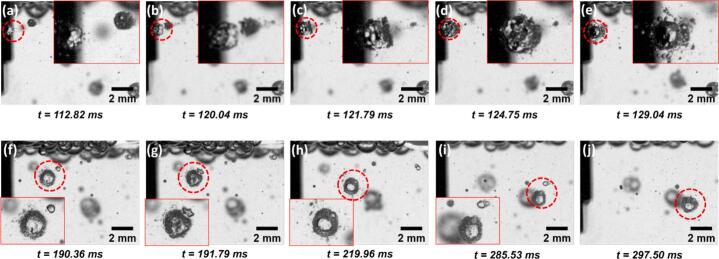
Bubble coalescence in TX-100 solution at 100 % CMC during UST. The inset images in the figures show zoomed-in images of the bubble cluster labelled by red circle with adjusted brightness and contrast. Time values represent the elapsed time after the onset of ultrasonication. (For interpretation of the references to colour in this figure legend, the reader is referred to the web version of this article.)
